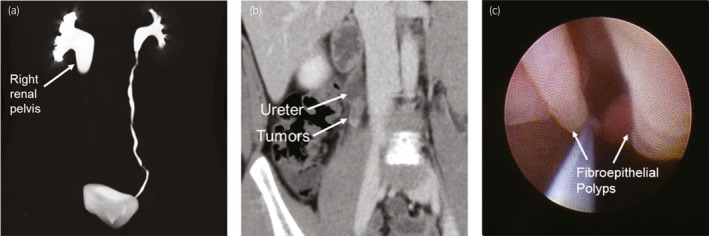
Enhanced computed tomography showing (a) grade 3 hydronephrosis with UPJ obstruction and (b) ureteral tumors at the right UPJ. (c) Diagnostic URS findings of metachronous UFEPs obstructing the right ureter. UFEP, ureteral fibroepithelial polyp; UPJ, ureteropelvic junction; URS, ureteroscopy.
